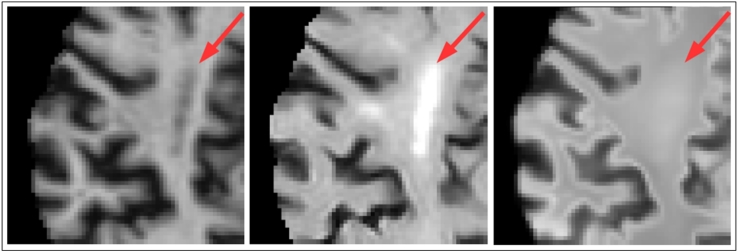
A case where a lesion is correctly synthesised as the same intensity as the surrounding WM. Left: *T*_1_-w image. Middle: FLAIR image. Right: Corresponding synthetic FLAIR image.
